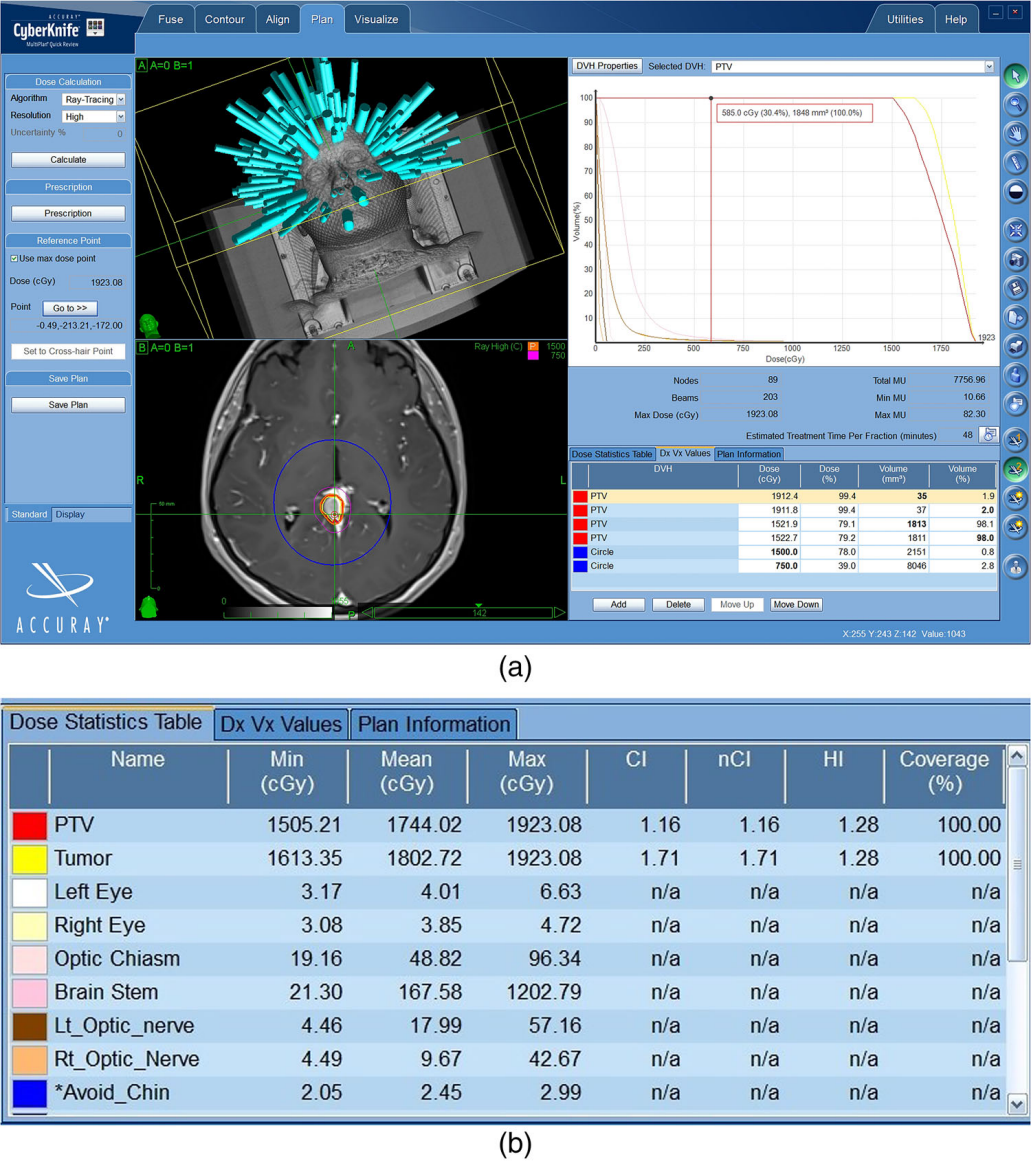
(a) The CyberKnife's treatment planning system (i.e., MultiPlan) with an example meningioma case, with a prescription dose of 1500 cGy, selected. The dose‐volume metrics tab (i.e., Dx Vx Values tab) is selected in the lower right corner. The isodose lines for the prescription dose (orange) and half the prescription dose (i.e., 750 cGy) (pink) are displayed in the MRI transverse view, along with the cylindrical structure (i.e., Circle structure) (blue), contoured into the plan to ultimately determine the GI. (b) Dose Statistics Table from the selected treatment plan.
